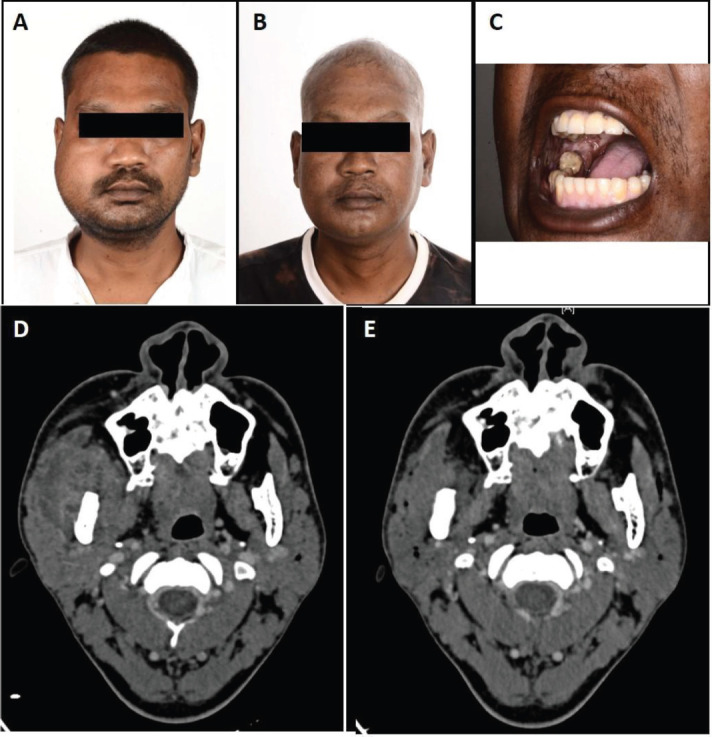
Clinico-radiological response after NACT. (a): Pre-chemotherapy image showing oedema above zygoma and fullness in infratemporal fossa. (b and c): Post-chemotherapy image showing near complete resolution of peri-tumoral oedema and buccal mucosa lesion, respectively. (d): Pre-chemotherapy CT image showing high ITF involvement. (e): Post-chemotherapy CT image showing significant reduction in tumour bulk and clearance of disease from high ITF.
